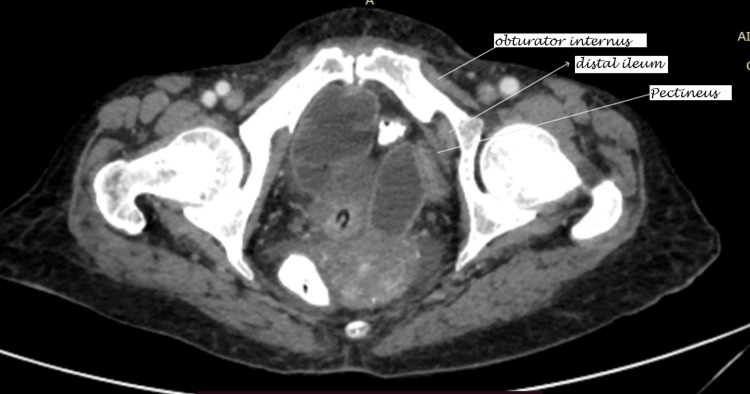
A CECT of the abdomen (axial view) shows an obturator hernia (distal ileum) with dilated small bowel loops. CECT: contrast-enhanced computed tomography
